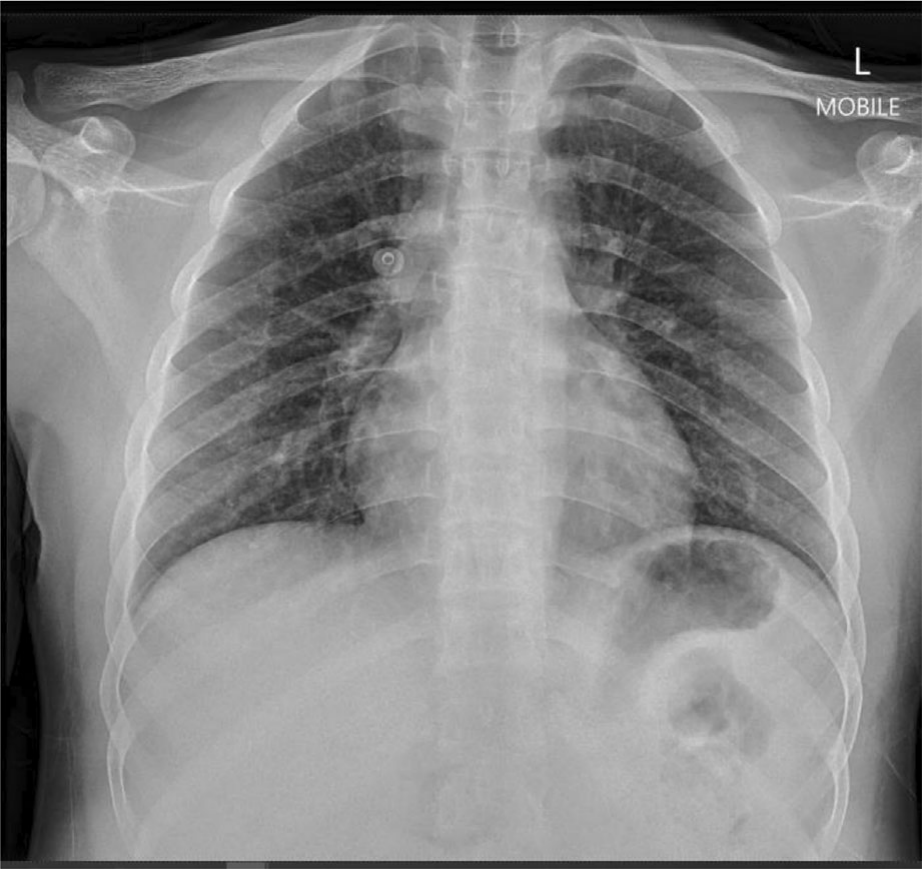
Chest radiograph on admission showing bilateral pulmonary infiltrates
